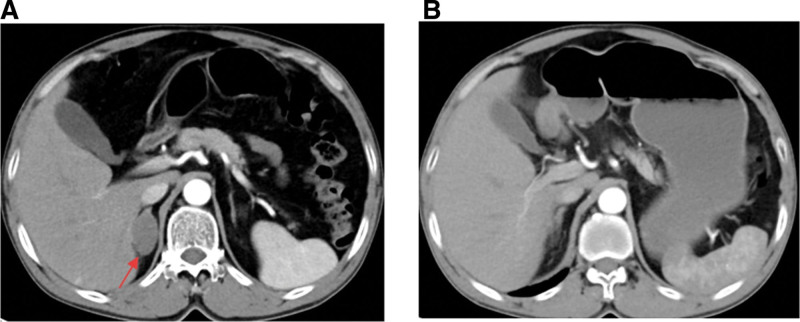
Abdominal CT images demonstrating the disappearance of the right adrenal gland metastasis (A and B). CT = computed tomography.
